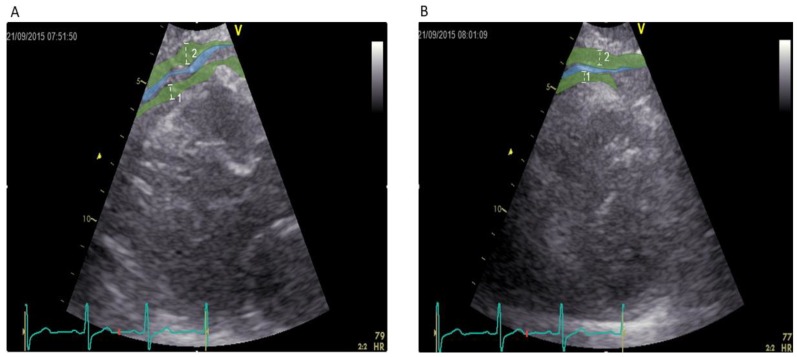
(A) Identification of tissues in parasternal long axis projection modified to focus on right ventricle (PLAX mod). Epicardial adipose tissue (EAT) thickness was identified as the maximum diameter (white line 1) of the hypoechogenic space between the myocardium of the right ventricle and the visceral pericardium (blue area). The area at the level of the aortic root is excluded from the measurement of EAT due to fact that EAT is physiologically present in this area. Paracardial adipose tissue (PAT) thickness was identified as the maximum diameter (white line 2) of the hypoechogenic space between the visceral pericardium (blue area) and the mediastinum. Both in the middle line along the midline of the ultrasound beam at end-systole; (B) Identification of tissues in parasternal short axis projection papillary muscle level (PSAX). Level of the PSAX was selected individually for every patient and is dependent on the identification point in the PLAX mod. EAT thickness was identified as the maximum diameter (white line 1) of the hypoechogenic space between the myocardium of the right ventricle and the visceral pericardium (blue area). The area at the level of the aortic root is excluded from the measurement of EAT due to fact that EAT is physiologically present in this area. PAT thickness was identified as the maximum diameter (white line 2) of the hypoechogenic space between the visceral pericardium (blue area) and the mediastinum. Both in the middle line along the midline of the ultrasound beam at end-systole.
